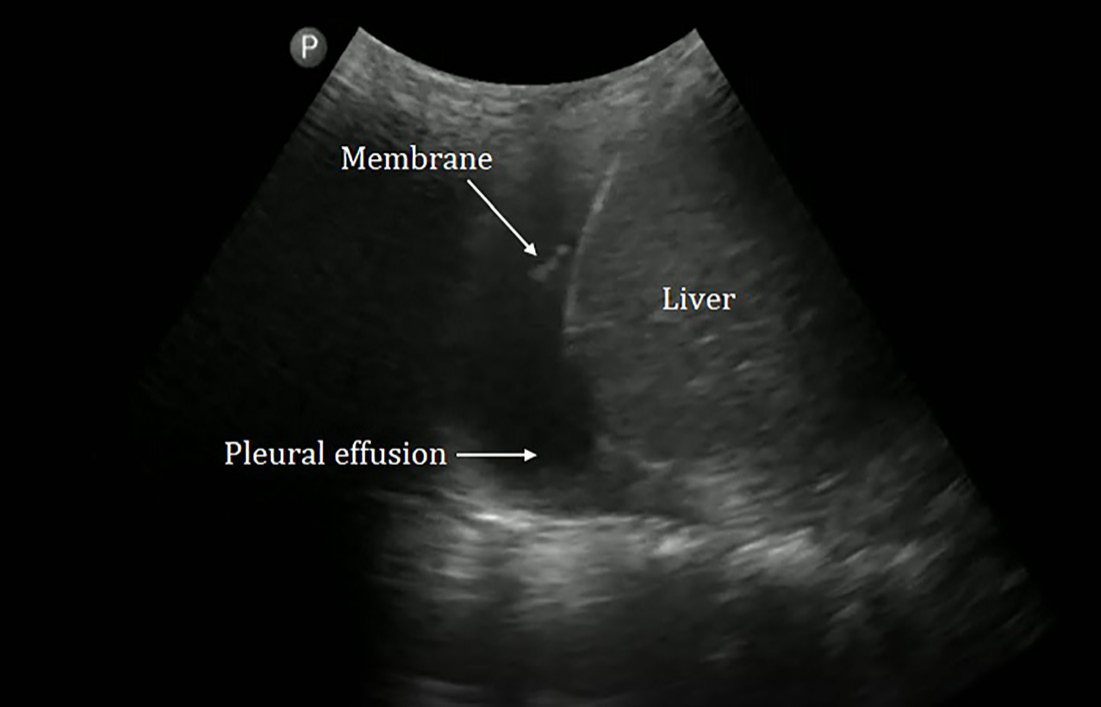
Point-of-care ultrasound of the right hemithorax showing a hyperechoic linear structure (suggestive of a membrane) floating in anechoic fluid (a pleural effusion).
